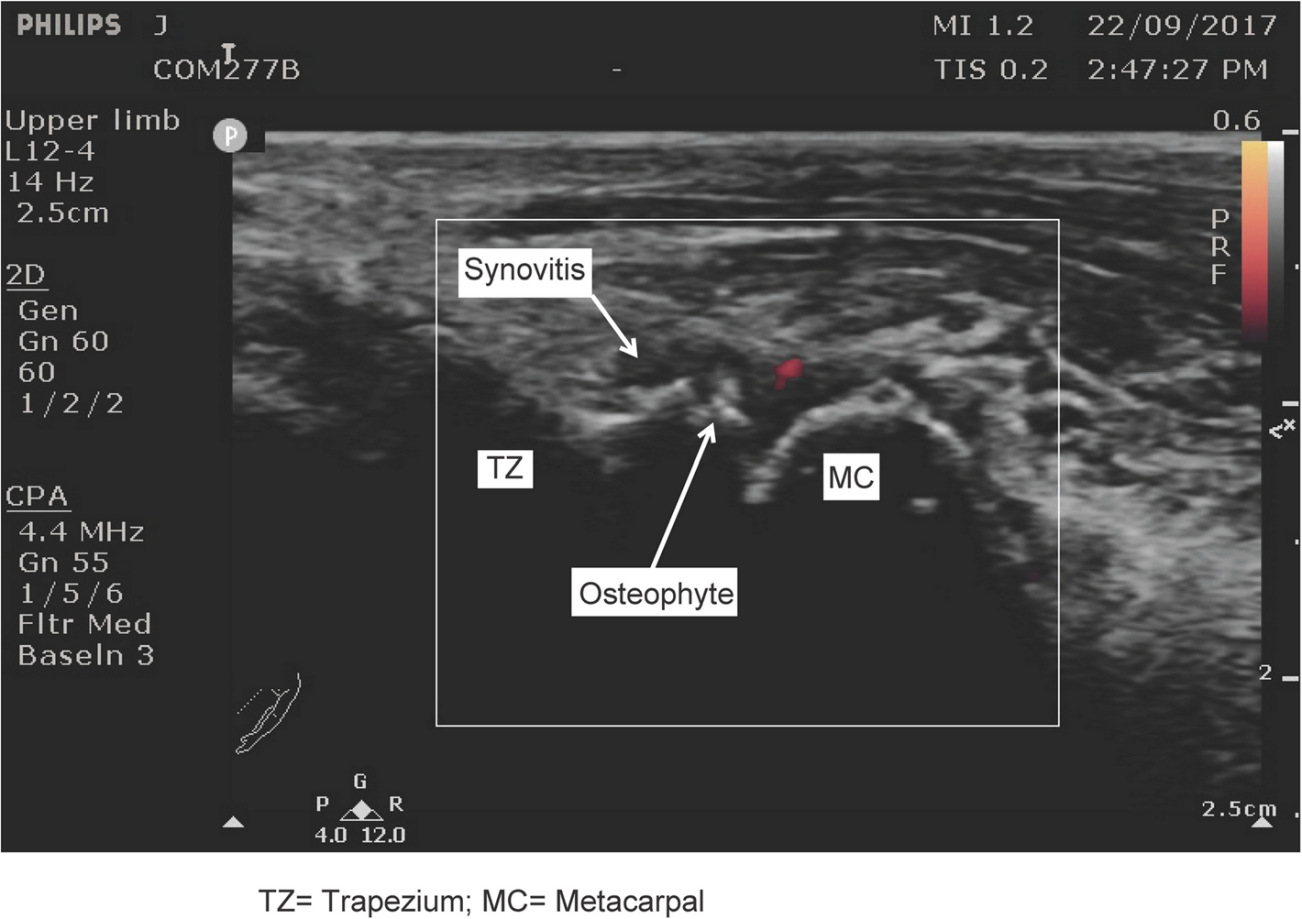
Power Doppler activity in thumb-base osteoarthritis. TZ = Trapezium; MC = Metacarpal
